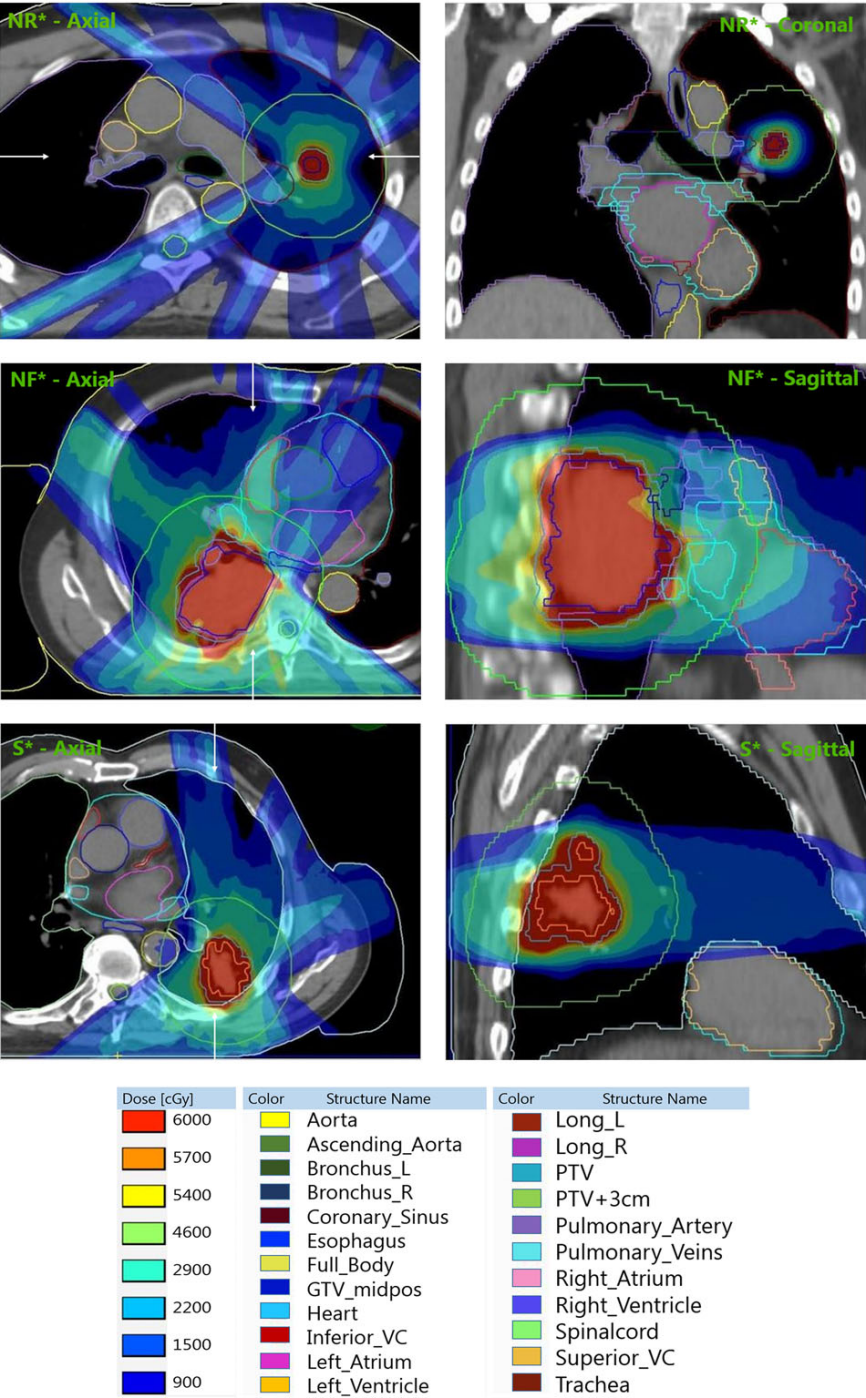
Examples of patient cases for cardiac sparing not required (NR*), not feasible (NF*), and successful (S*), respectively. Computed tomography (CT) scans are shown with a window setting of 500 Hounsfield units (HU) and level of 50 HU.
